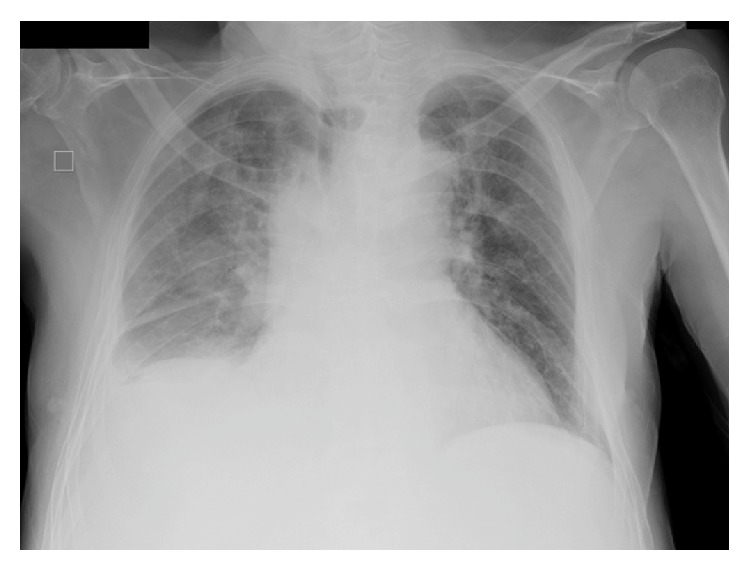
Chest X-ray on admission. Bilateral alveolar infiltrates with associated right pleural effusion.
